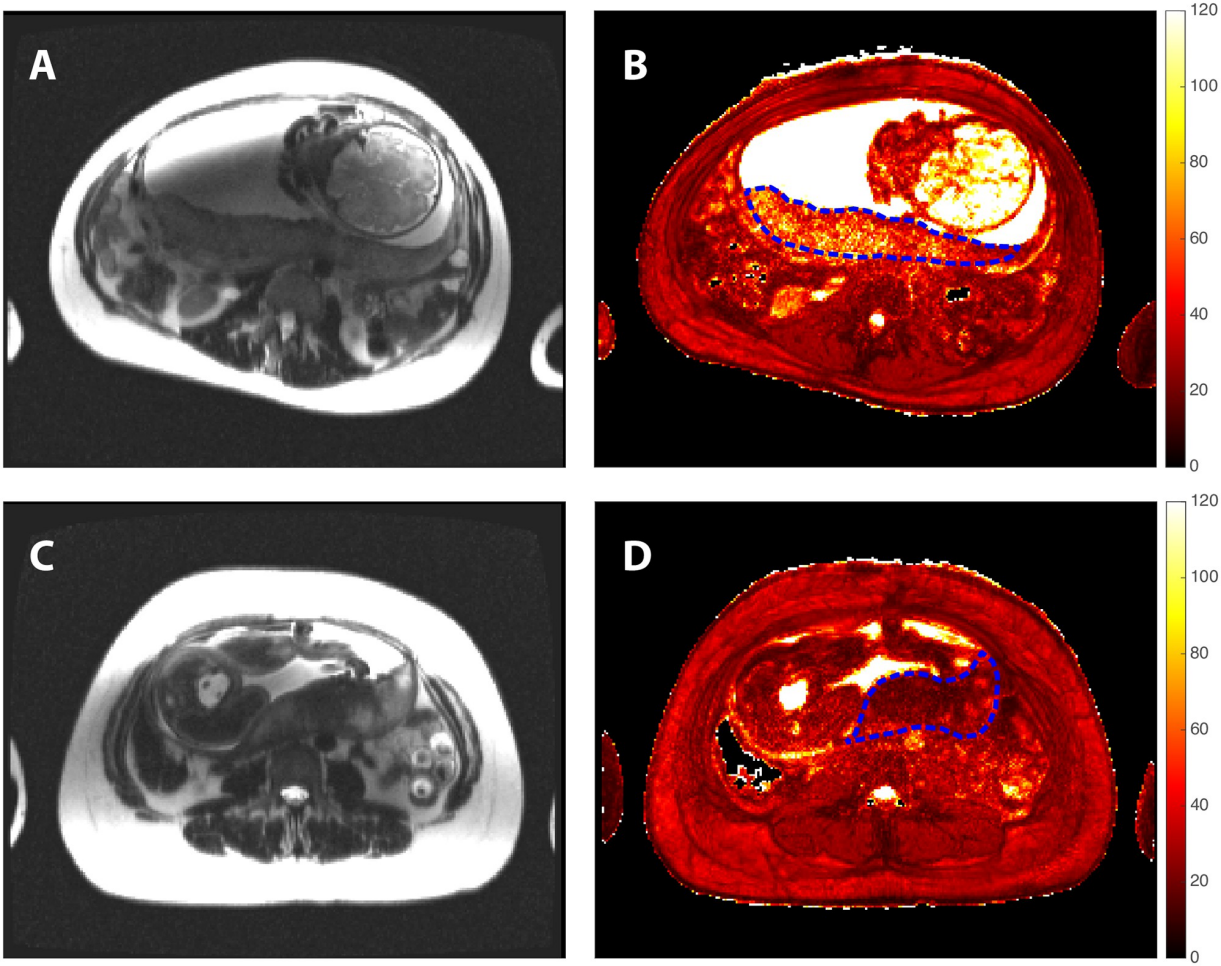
Comparison of anatomic magnetic resonance imaging and placental T2* mapping in uncomplicated normal and primary adverse pregnancies. T2-weighted HASTE MRI (left column) and placental T2* maps (right column) are shown for an uncomplicated normal pregnancy at 232 days gestation (top row, panels A & B) and for a primary adverse pregnancy at 235 days gestation presenting with severe preeclampsia (bottom row, panels C & D). The placenta is indicated by the dashed blue outlines overlaid on the T2* maps.
